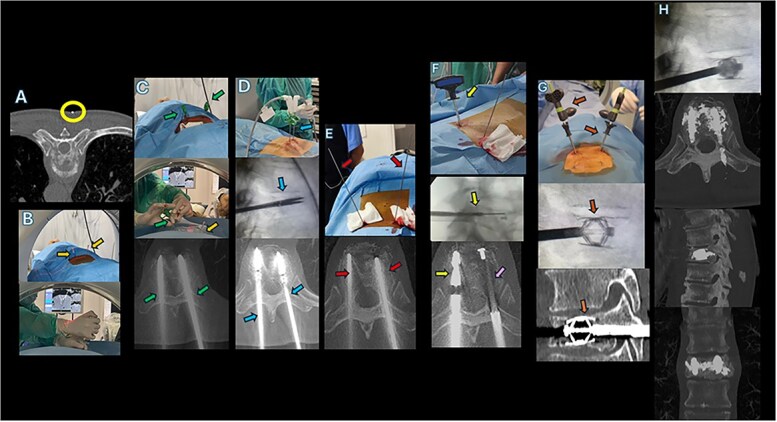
2A: Marker placement. Yellow circle: Metallic markers are inserted into the vertebra at predetermined positions after measuring from the midline to the lateral edge of the vertebra, verified by an axial CT scan. 1B: Needle placement and Anesthesia Orange arrows: Local anesthesia in periosteum (bupivacaine). 2C: Needle insertion for Vertebroplasty:Green arrows: 11-gauge vertebroplasty needles are inserted transpedicular, guided under real-time fluoroscopy to reach the anterior third of the vertebral body with final CT scan check. 2D: Microwave ablation (MWA): Blue arrows: A 20 cm microwave antenna is inserted through the vertebroplasty needle, ensuring the antenna covers the entire lesion. Ablation is performed using a 2.45 GHz microwave generator to target the tumor. CT scan is crucial to obtain a correct measurement of ablation volume to avoid complications. 2E: Kirschner wire insertion red arrows: Kirschner wire is inserted into the vertebral body after removing the vertebroplasty needle’s inner cannula, advancing under fluoroscopic guidance to the anterior third of the vertebral body. CT scan is crucial to rule out any misalignment or to assess if and how the creation of the subsequent channels can be improved. 2F: Drilling and Bone Canal preparation: Yellow arrow: A drill is advanced under fluoroscopic guidance along the Kirschner wire. Pink arrow: An acrylic plug is left in place after the drill is removed, allowing preparation for the subsequent implant channel preparation. 2G: SpineJack® implantation:Brown arrows: Two SpineJack® expandable titanium implants are inserted and gradually deployed under fluoroscopic guidance, restoring vertebral height and reducing kyphosis. 2H: Post-procedural fluoroscopy image and multiplanar CT scan: Post cementation lateral fluoroscopic imaging and CT scan, with multiplanar (MPR) reconstruction scan to assess implant placement, cement distribution, and symmetry of vertebral body expansion. This scan also helps identify potential complications such as cement leakage.
